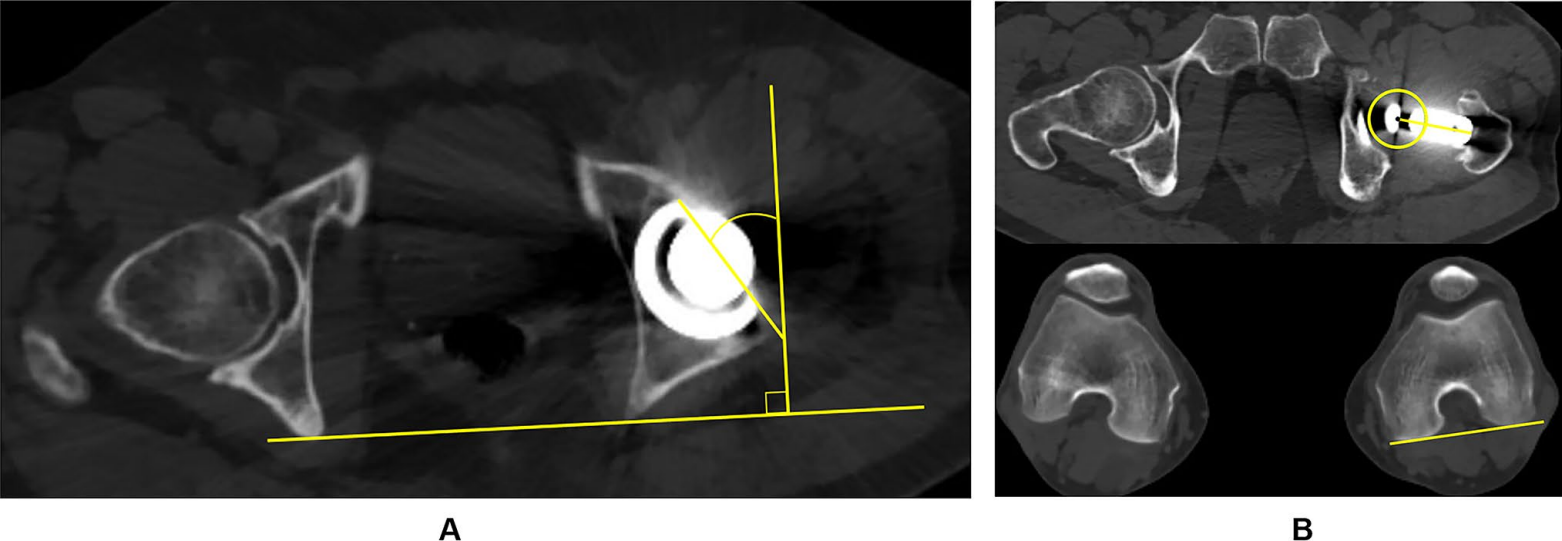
Axial computed tomography (CT) image demonstrating the method used to measure the A acetabular anteversion as the line connecting the most anterior and posterior point of the acetabular cup and a reference line drawn perpendicular to a line between the most posterior pelvic margins and B femoral anteversion defined as the angle between a line connecting the center of the femoral head to the center of the base of the femoral stem neck and the line connecting the posterior aspect of the medial and lateral femoral condyles
